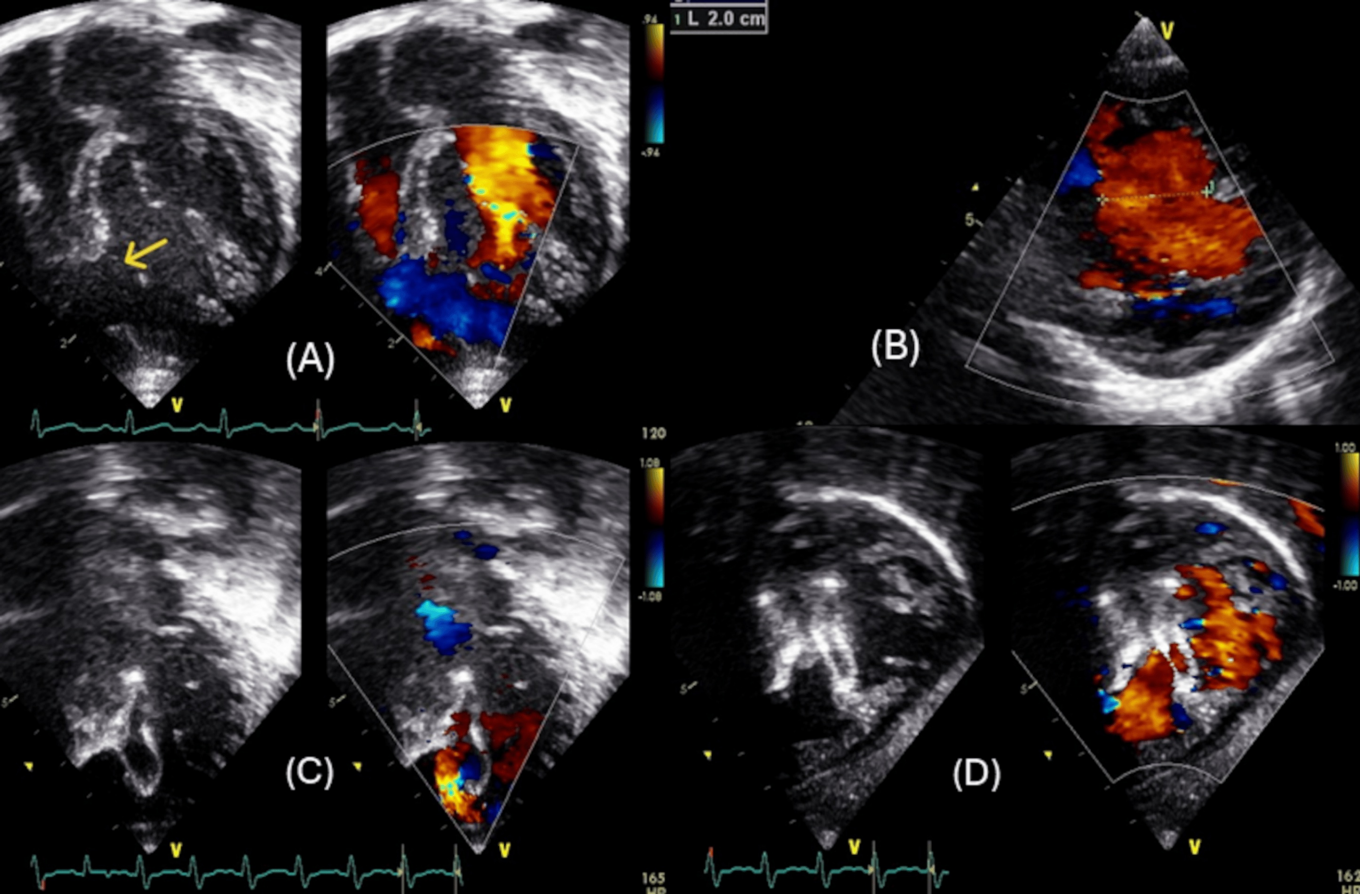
Case 2 echocardiogram images (A)&(B) Ventricular septal defect (VSD) assessment on echocardiogram (apical four-chamber and parasternal short axis views). (C) Device immediately after deployment (apical four-chamber view). (D) Device after 12 weeks of deployment (modified subcostal coronal axis view). The yellow arrow shows the VSD.
